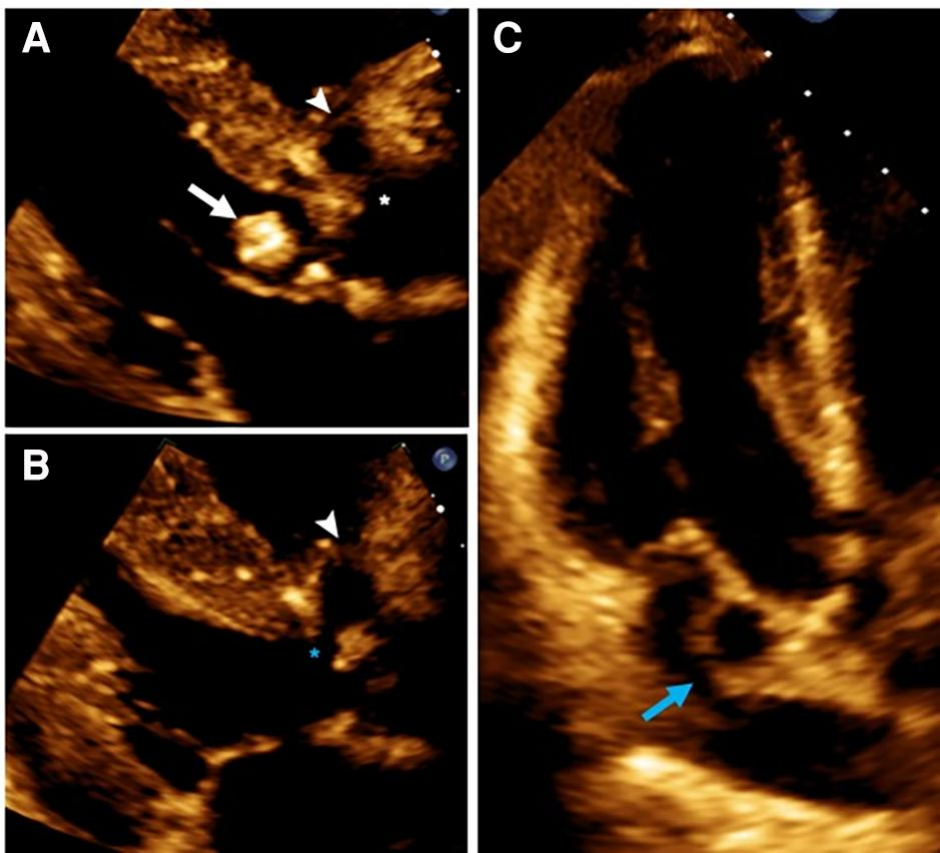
Transthoracic echocardiography. (*A*) Parasternal Long Axis View (PLAX) during diastole showing a mobile vegetation on aortic valve (*white arrow*) and anterior pseudoaneurysm (*white arrow head*), with aortic fistulization (*white asterisk*). (*B*) PLAX showing during systole anterior pseudoaneurysm and fistulization to left ventricular outflow tract (*blue asterisk*). (*C*) Three-chamber view during systole demonstrating pseudoaneurysm of the mitral–aortic intervalvular fibrosa (*blue arrow*).
